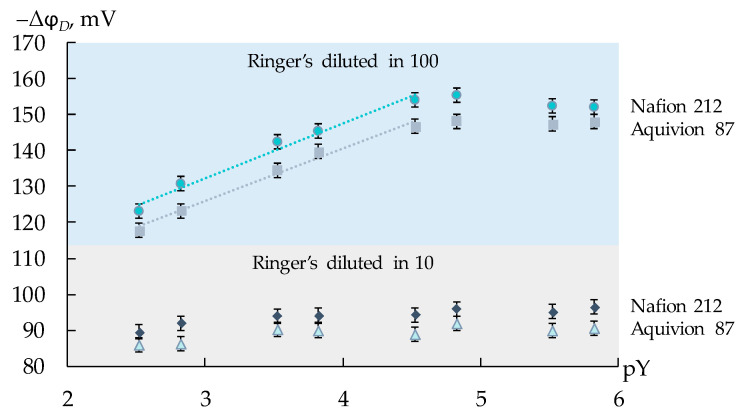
Dependence of the DP-sensor response on the negative decimal logarithm of the total concentration of AM^+/±^, CT^+/±^, and LS^+^ ions (pY) in artificial saliva solutions diluted 10 and 100 times.
